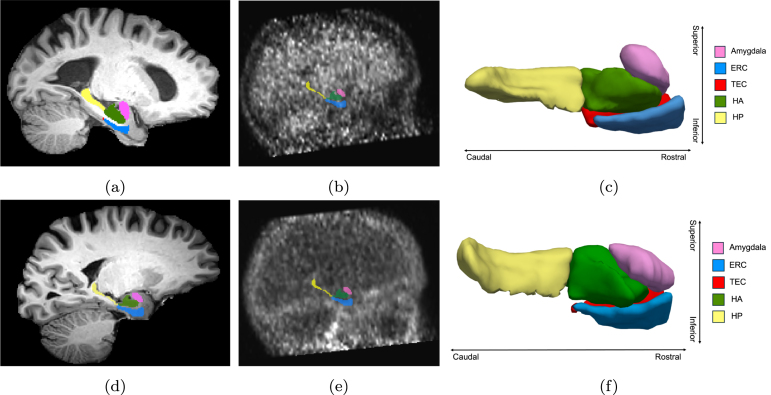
Sagittal sections of T1-weighted image for a subject in (a) ADNI and (d) BIOCARD with (b) tau and (e) amyloid PET images. 3D segmentation of a subject in ADNI (c) and BIOCARD (f) dataset.
